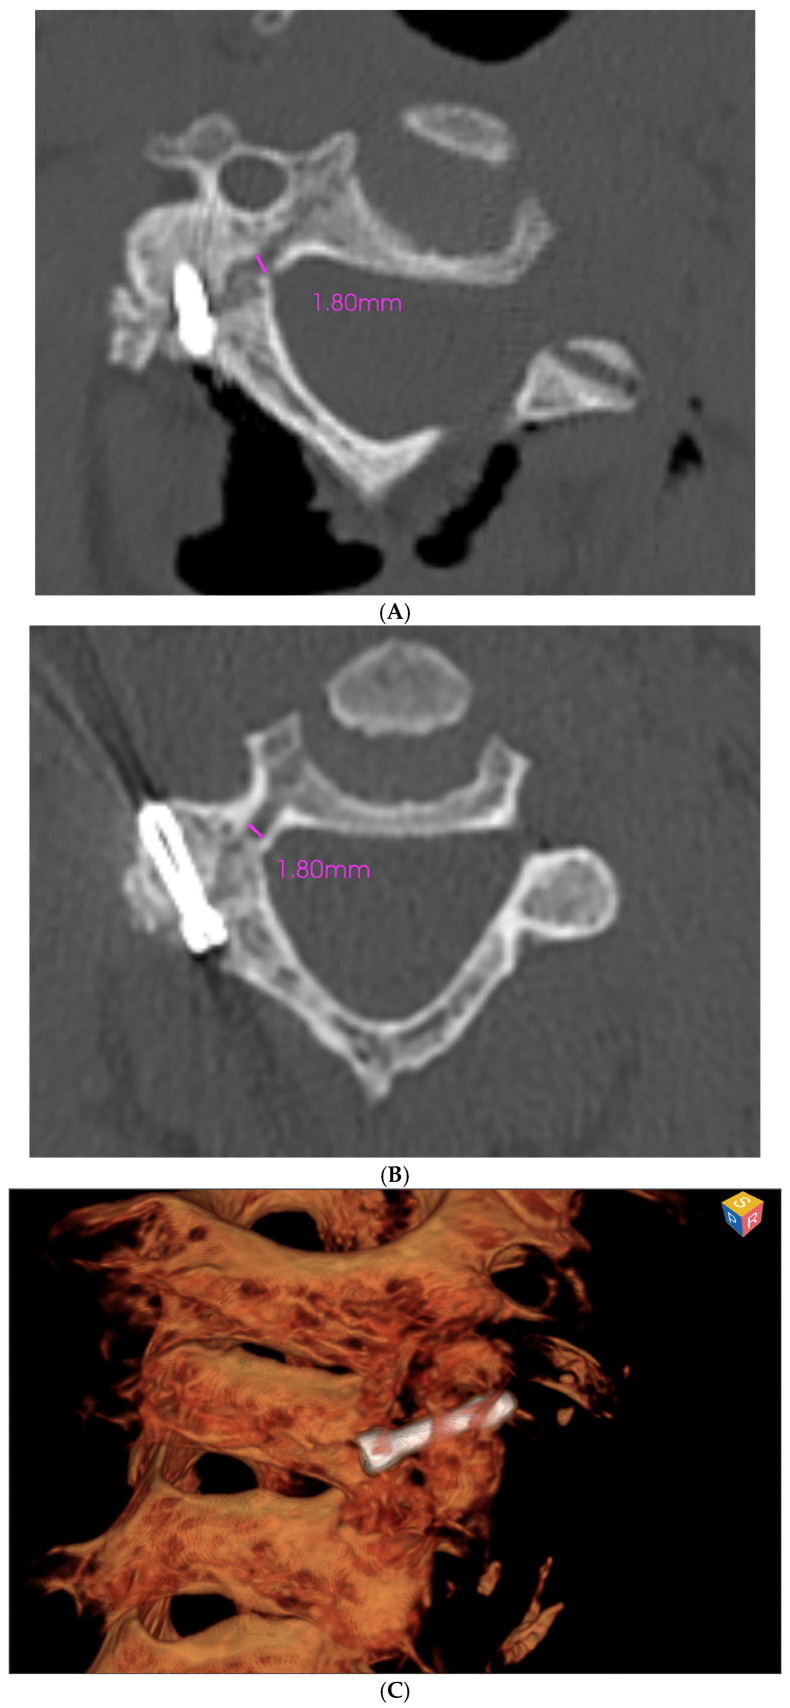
(A) Immediate postoperative scan highlighting fracture diastasis reduction and correct alignment of the bone heads as well as of the Herbert screw without violation of the vertebral artery canal. (B,C) CT scan and 3D reconstruction 3 months after the procedure, documenting bone fusion.
